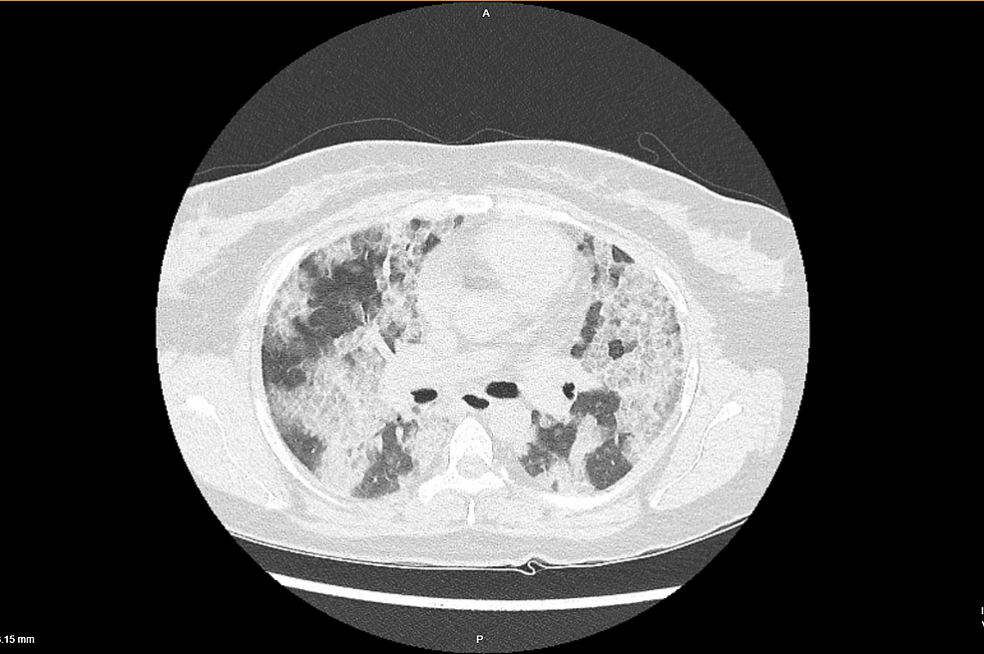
HRCT chest transverse section (Crazy-Paving pattern and ground-glass opacities). HRCT: high-resolution computed tomography.
